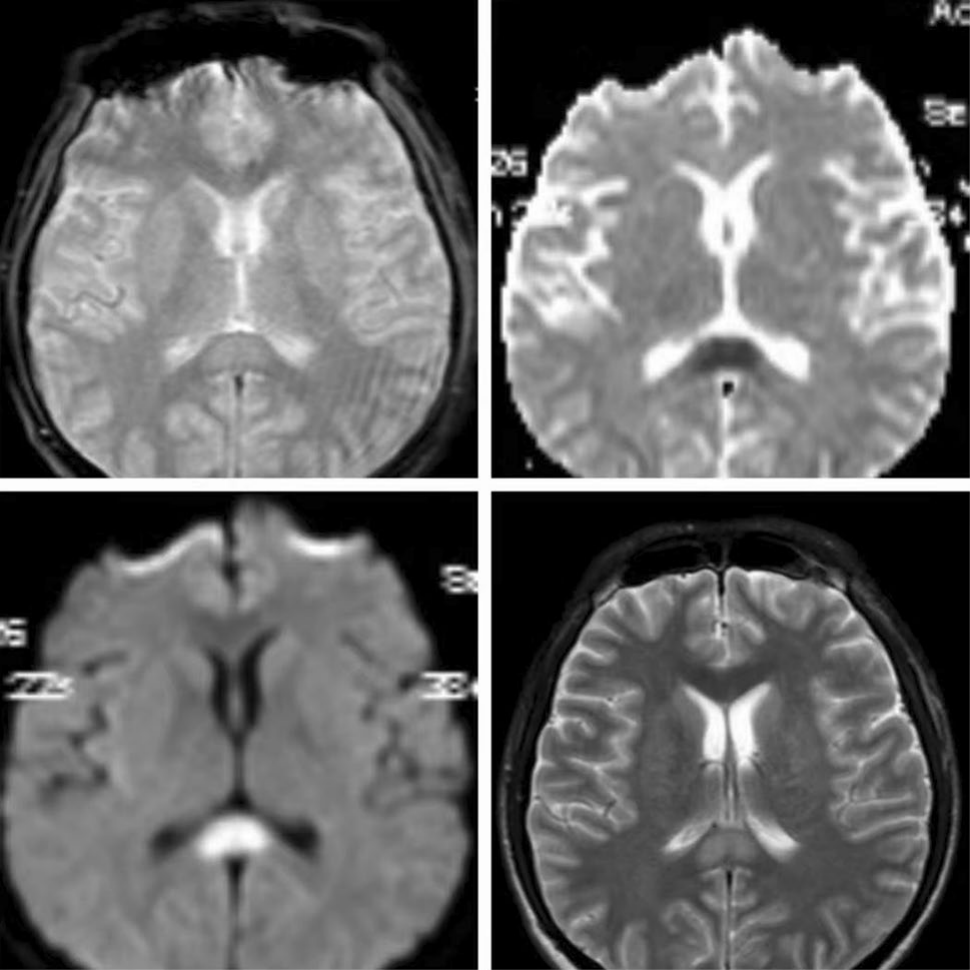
MRI of case 14: abnormal signal of the corpus callosum visible (note: two other patients had similar signal abnormalities in the corpus callosum)
